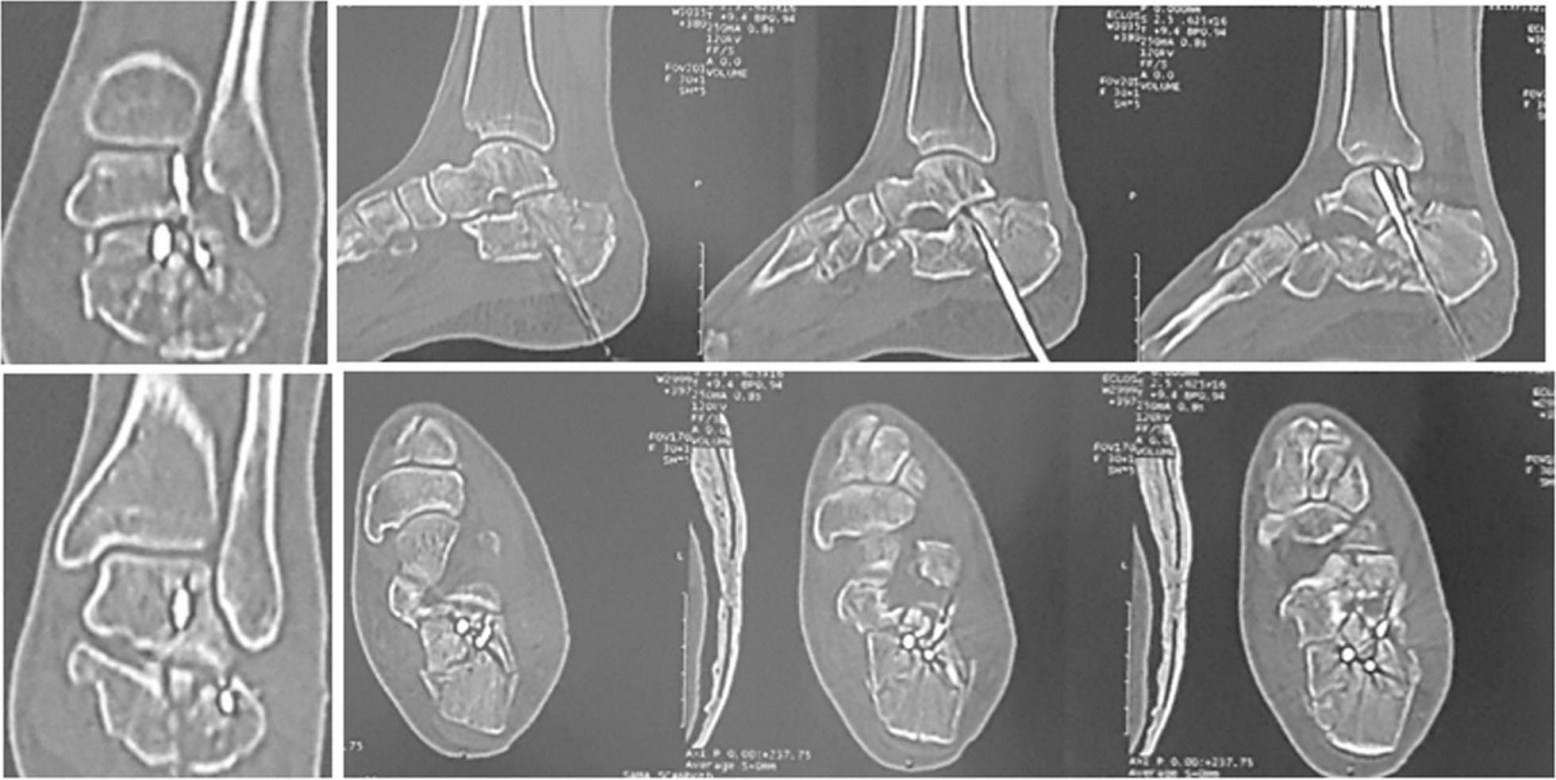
Postoperative CT for evaluating the articular surface reduction
